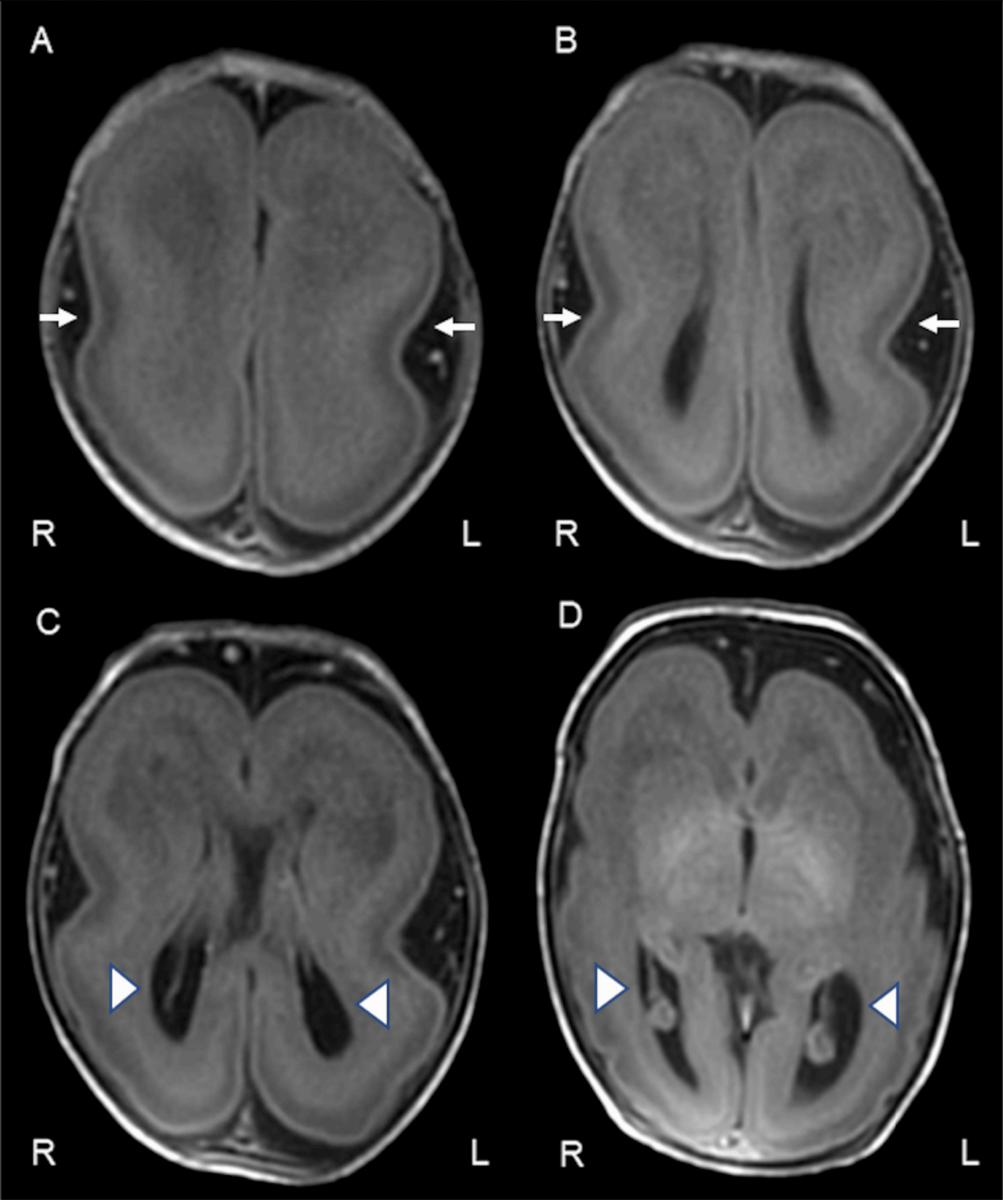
Axial T1-weighted magnetic resonance imaging on day seven of life R: right, L: left. Axial T1-weighted MRI scans on day seven of life demonstrating characteristic features of classical lissencephaly.
